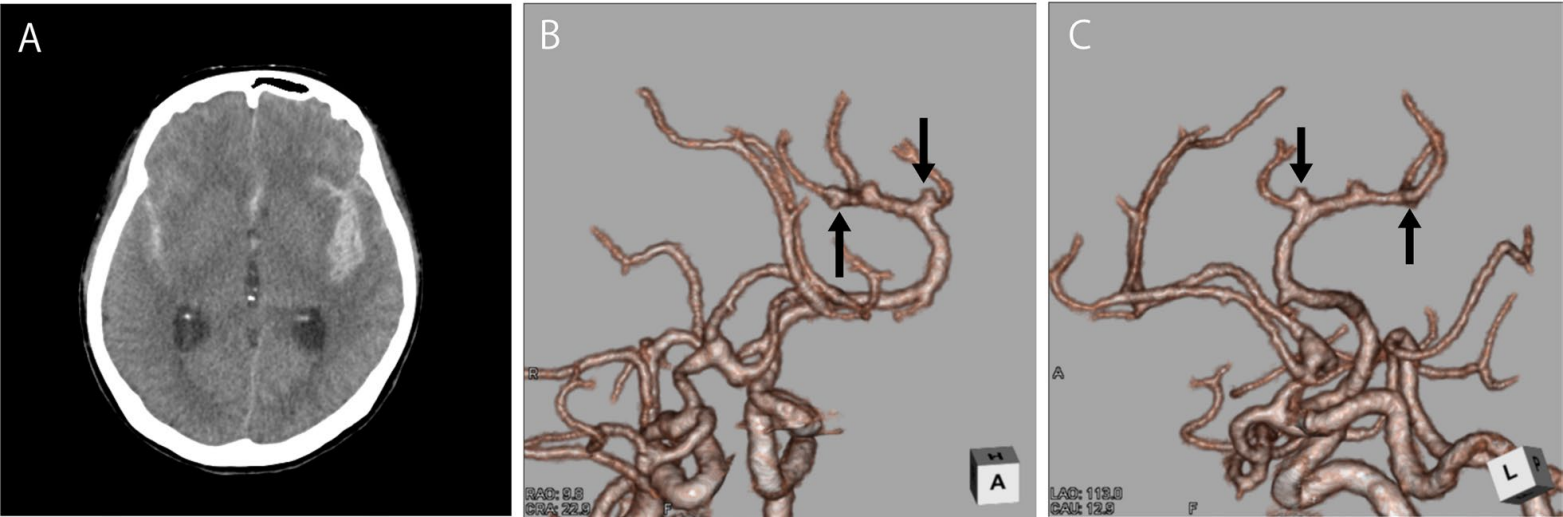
Subarachnoid hemorrhage due to the rupture of intracranial aneurysms. The patient was transported to our hospital because of acute headache and disturbance of consciousness. A Computed tomography (CT) showing subarachnoid hemorrhage. B (anterior view), C (lateral view), CT angiography revealing multiple intracranial aneurysms (< 2 mm) (arrow). Because brain magnetic resonance imaging performed in the previous year did not find aneurysms at the same site, she was diagnosed with rupture of a *de novo* aneurysm
